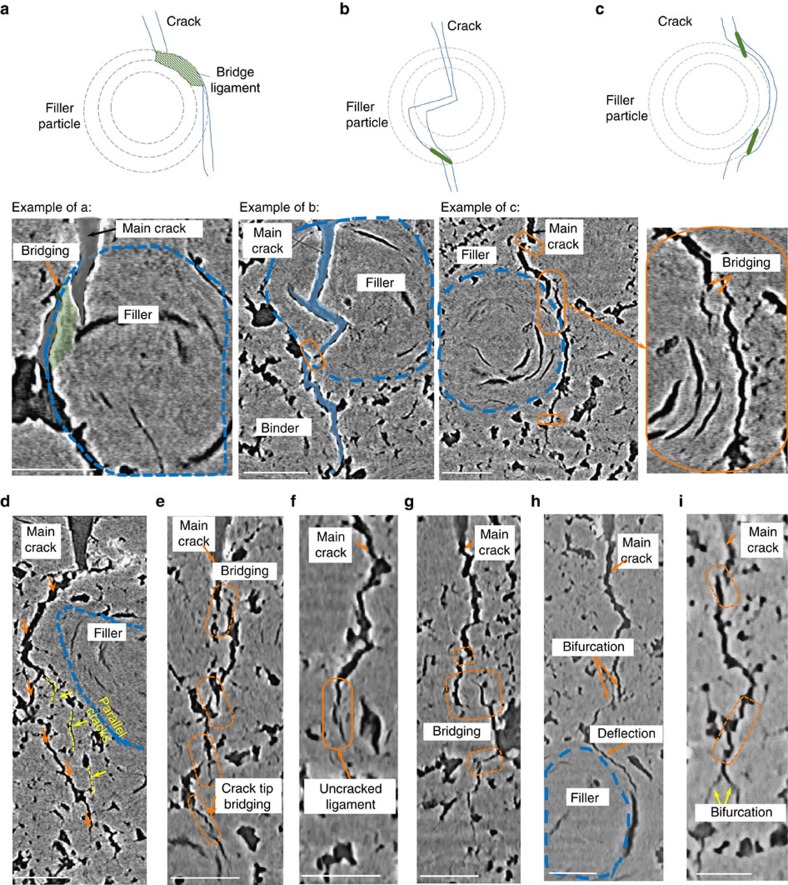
Computed tomography shows generation of extrinsic toughening mechanisms. In Gilsocarbon graphite, crack deflection and crack bridging occurs at 20 °C when the main crack interacts with a filler particle in three ways: (a) as the main crack grows through the side of a filler particle, the material between the pores around the particle and circumferential crack inside the particle tend to form a bridge site; (b) the crack enters through the centre of a particle and is deflected by the central calcination cracks such that bridges form when it exits the particle to join the matrix; (c) the crack misses the main part of the particle but bridges still form around the filler particle due to the change in crack path; (d) example of parallel crack coexisting with the main crack at 20 °C. Examples at 1,000 °C of (e) the discontinuous main crack interrupted by various bridging sites and increased number of small bridges at the crack tip, combined with uncracked-ligament shielding; (f) uncracked-ligament bridging near the crack tip; (g) the combination of very large ligament bridging and very small ones as the crack path changes direction; (h) crack deflection at filler particles; and (i) with consequent bifurcation occurring at the crack tip. All scale bars, 100 μm.
